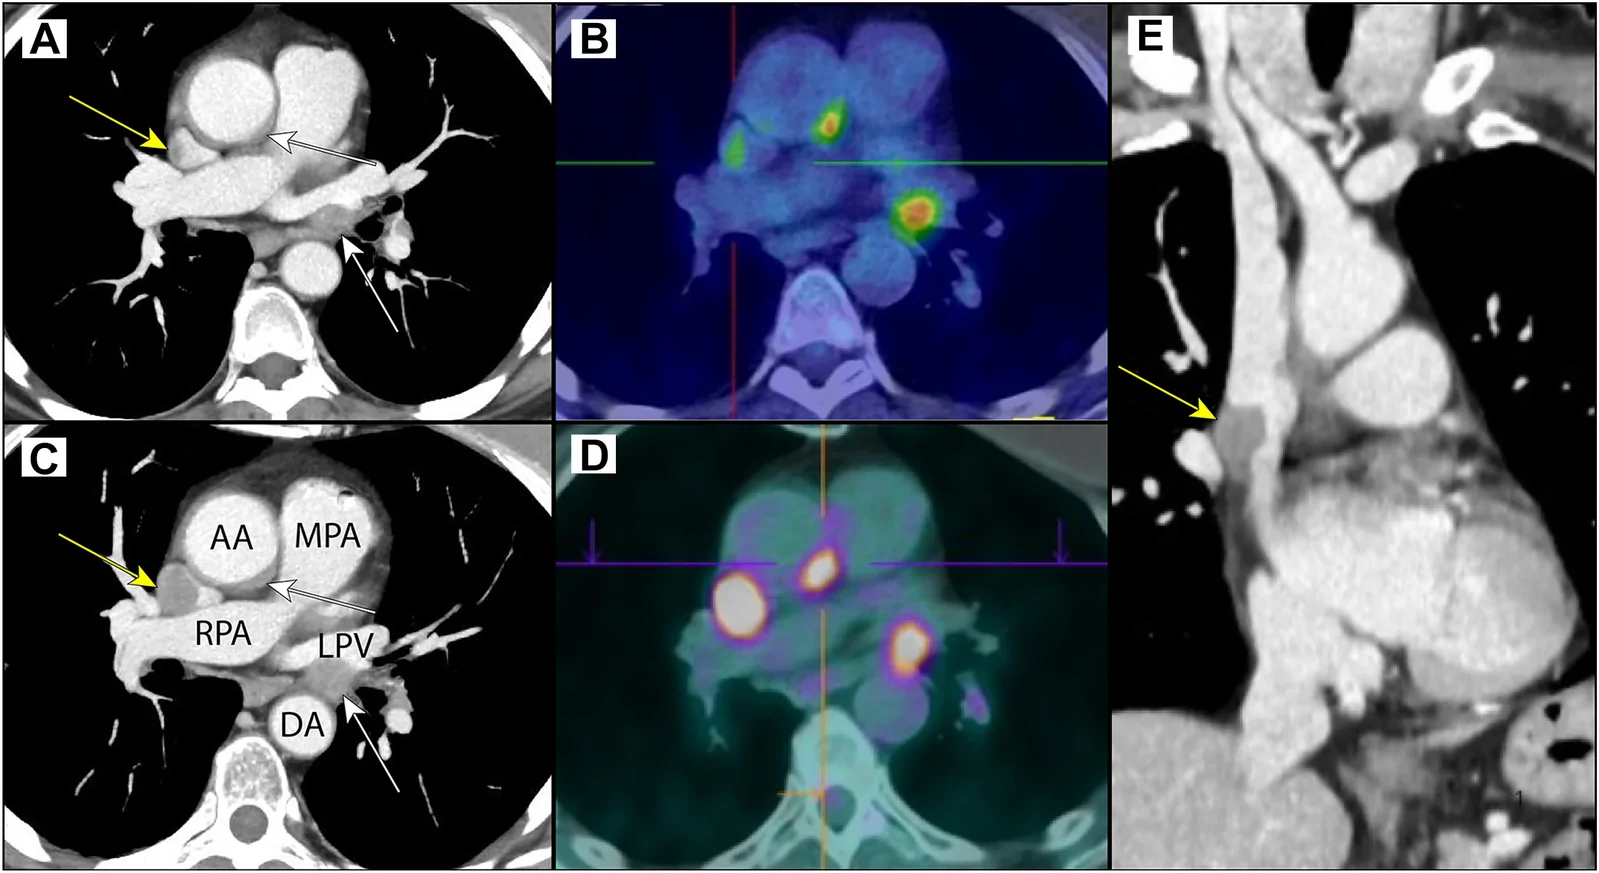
Computed tomography (CT) and ^18^F-fluorodeoxyglucose positron emission tomography (^18^F-FDG PET) images. (A) Baseline axial contrast-enhanced CT scan showing the nodule within the wall of the superior vena cava (yellow arrow) and additional nodules in the left atrial wall and at the lower margin of the left inferior pulmonary vein (white arrows). (B) Baseline ^18^F-FDG PET scan demonstrating initial modest hypermetabolic activity of the nodule. (C) Follow-up axial contrast-enhanced CT scan revealing increased size of the nodules (yellow arrow). Again, the white arrows show additional nodules in the left atrial wall and at the lower margin of the left inferior pulmonary vein. (AA, ascending aorta; DA, descending aorta; LPV, left pulmonary vein; MPA, main pulmonary artery; RPA, right pulmonary artery.) (D) Follow-up ^18^F-FDG PET scan showing marked increased metabolic activity. (E) Coronal CT scan depicting invasion of the superior vena cava by the nodule (yellow arrow).
